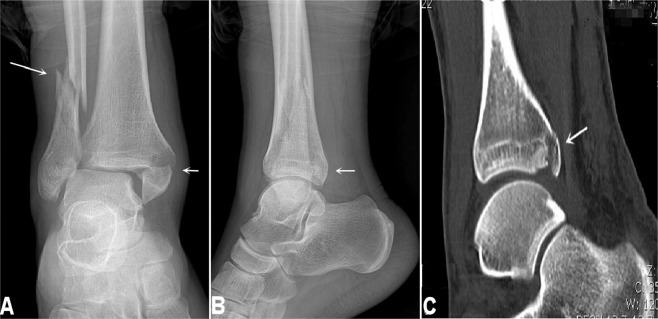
Medial malleolus fracture and high-location fibular fracture. (A). The medial malleolus fracture (short arrow) and the fibular fracture (long arrow) are shown. (B). Lateral radiograph shows inhomogeneous density at the posterior malleolus, suggesting possible fracture at this site. (C). Computed tomographic scan confirms the posterior malleolus fracture (arrow). This is stage 4 of the pronation-external rotation type of the Lauge-Hansen classification. The medial malleolus fracture line is transverse. The fibular fracture is oblique or spiral and is frequently located above the inferior tibiofibular joint level.
